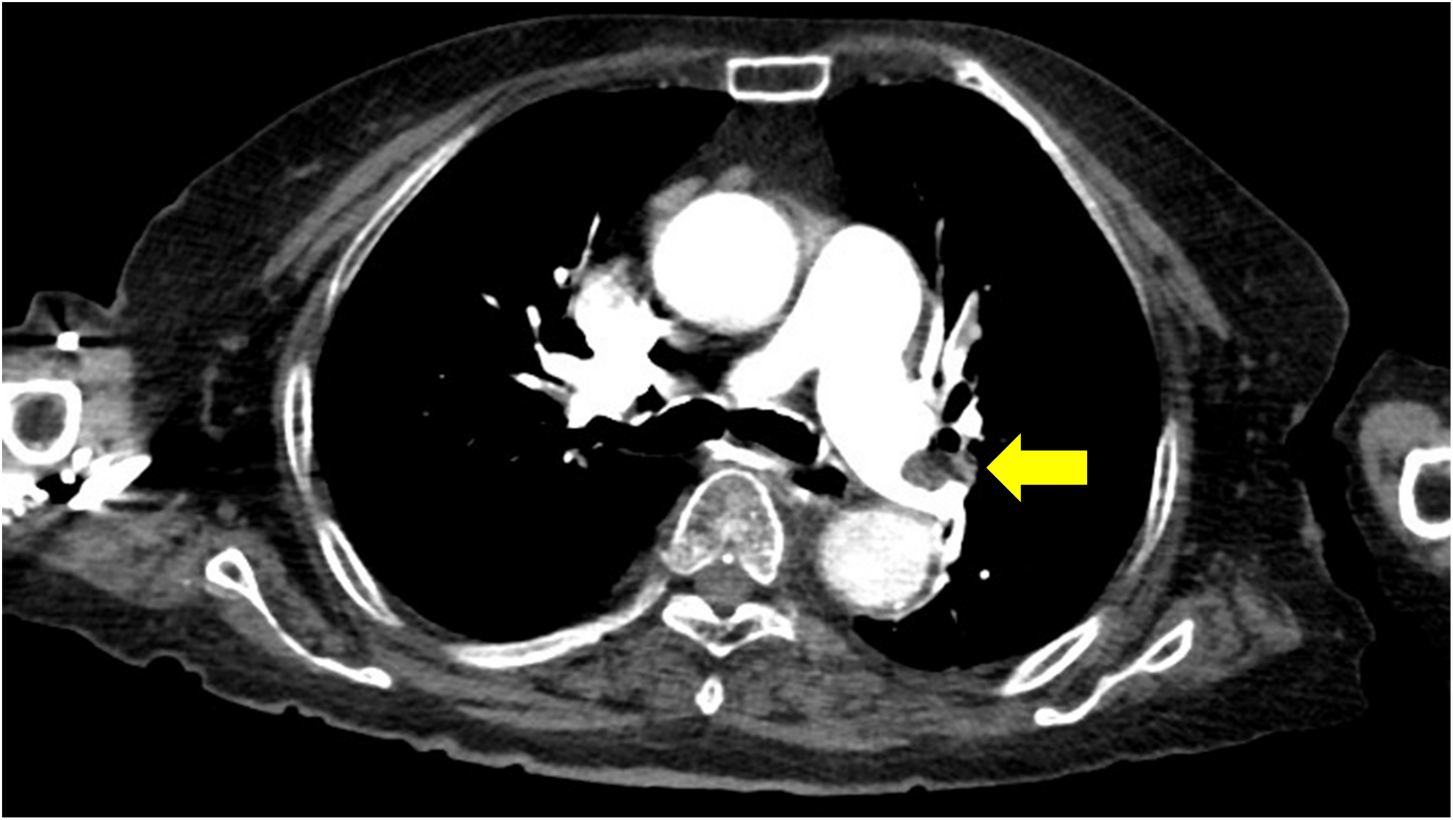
Computed tomographic pulmonary angiography indicating pulmonary emboli in the distal left main pulmonary artery.
